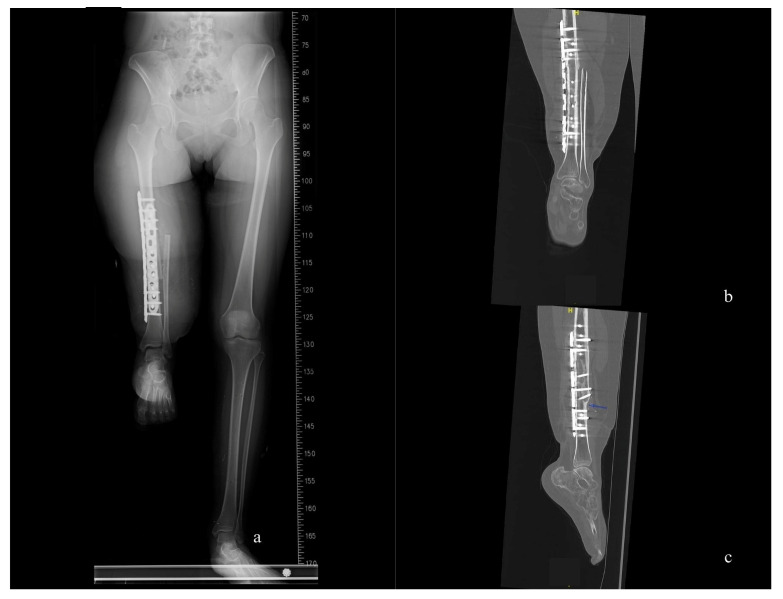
The position of the right “new knee” is appropriate. (a) Whole-leg axis radiographs at the two-months follow-up. (b,c) CT scans were conducted at the twelve-month follow-up, revealing complete healing of the bone union site and a straight alignment of the leg axis in coronal and sagittal view.
